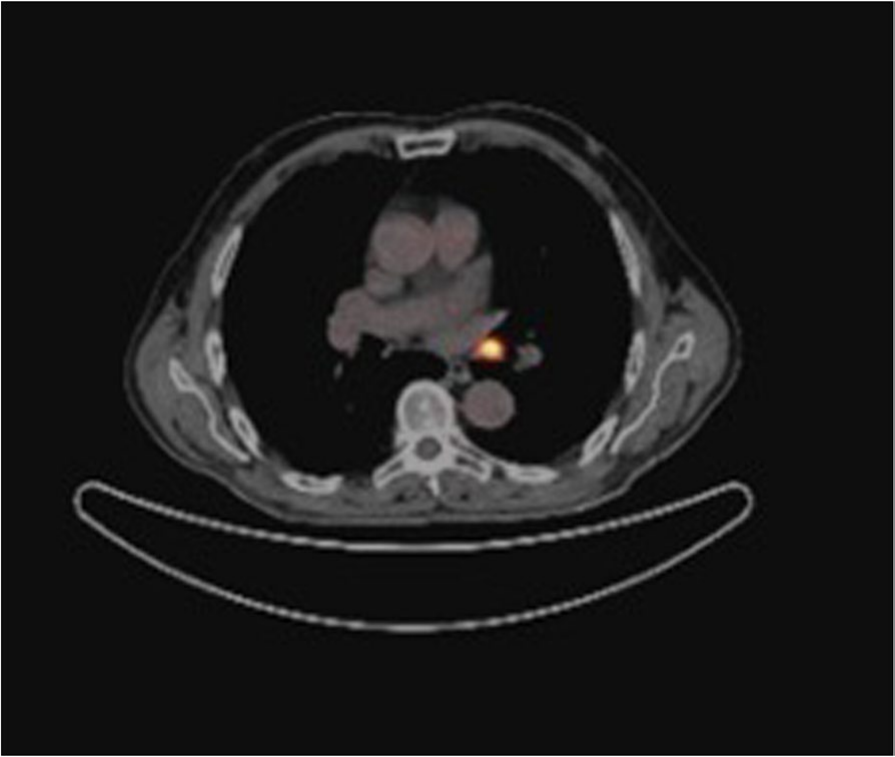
18FDG- PET/TC scan performed in February 2021 showed the increase of both dimension (12 mm ex 6 mm) and FDG-uptake (SUVmax = 27.2 ex 8.6) of the known lesion at the left inferior lobar bronchus
